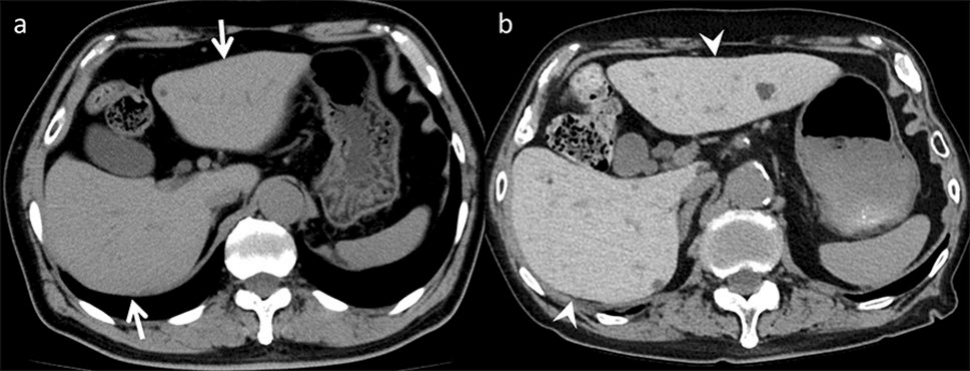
Amiodarone deposition in the liver of an 82-year-old man with ventricular tachycardia (VT), who was undergoing amiodarone therapy for VT. a Unenhanced computed tomography (CT) demonstrates normal liver attenuation (CT value, 57 HU) before amiodarone therapy (arrows). b Increased liver attenuation (97 HU) is observed on unenhanced CT performed nine years after amiodarone initiation (arrowheads). No abnormal liver function or hepatitis was indicated by hematological data or symptoms. It is difficult to differentiate amiodarone deposition from iron overload on a single image alone
